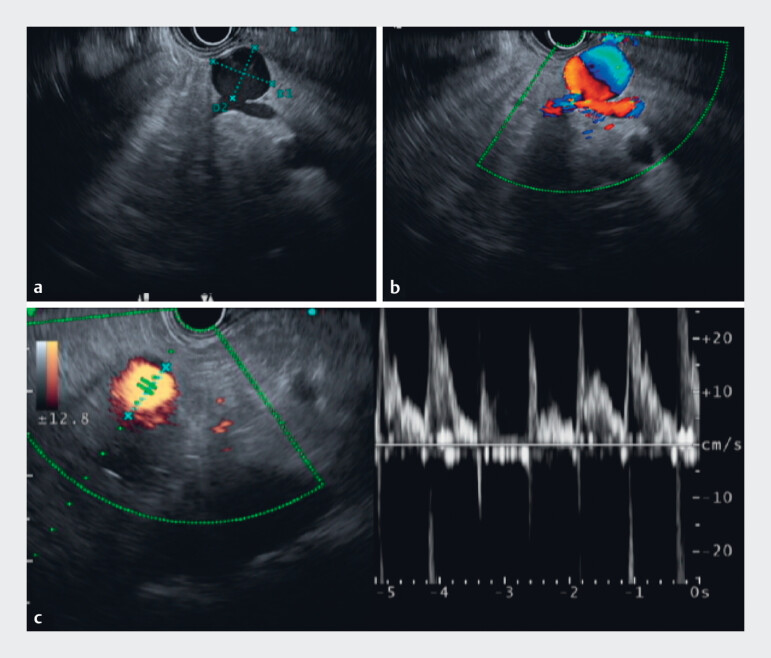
An endoscopic ultrasound (EUS) image utilizing a convex-type ultrasound for this patient. The EUS revealed a well-circumscribed, 18-mm anechoic lesion within the pancreatic head.
